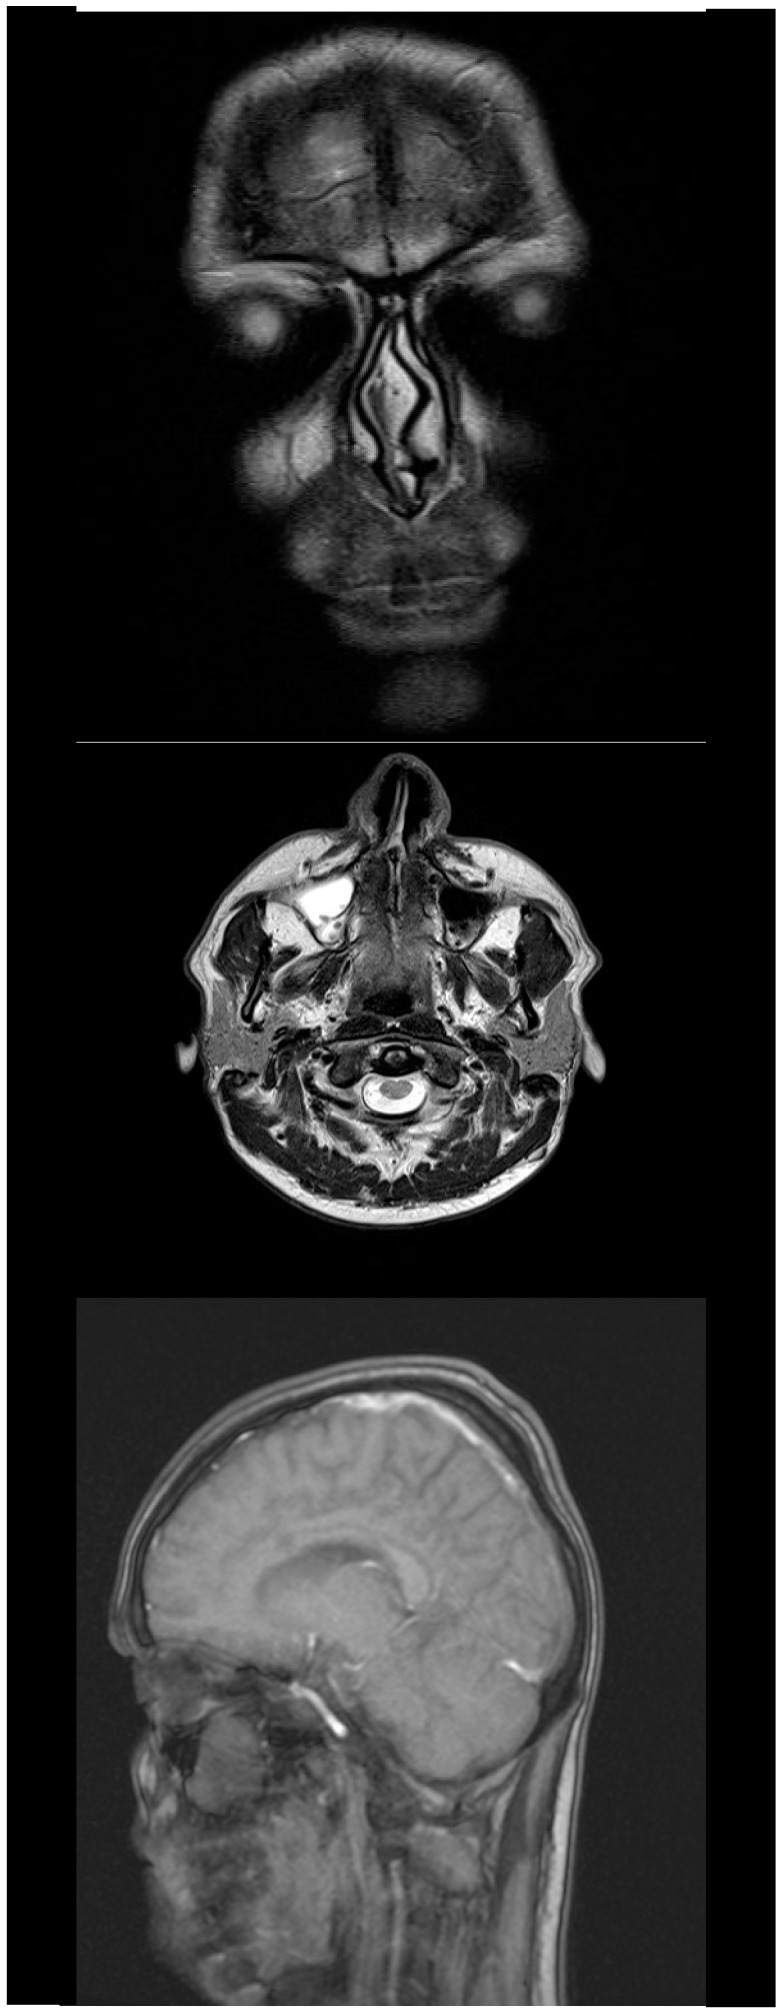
Cerebral MRI showing active lesions
